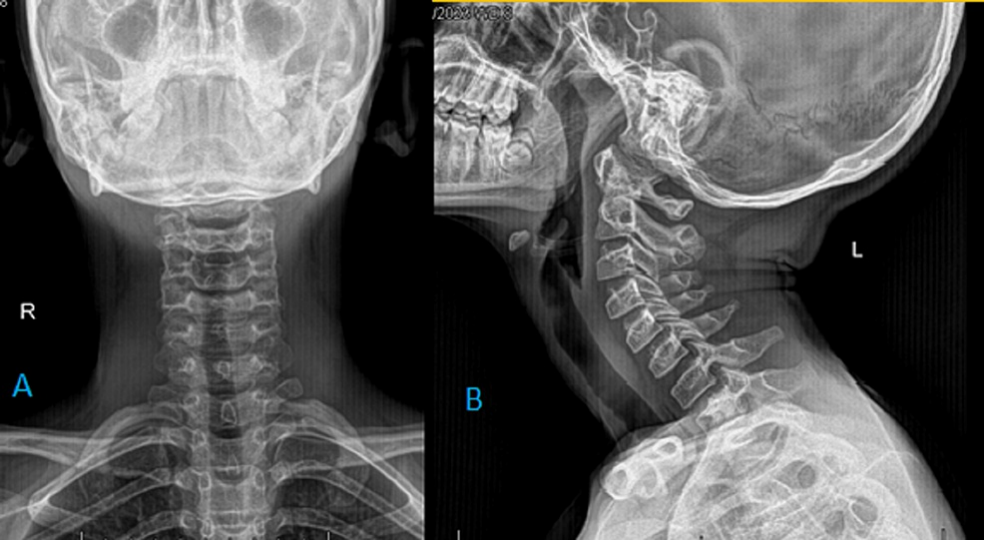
Follow up radiographs of neck AP (A) and lateral (B) views Follow up  radiographs of the neck obtained two weeks after discharge confirms complete resolution of air lucencies
